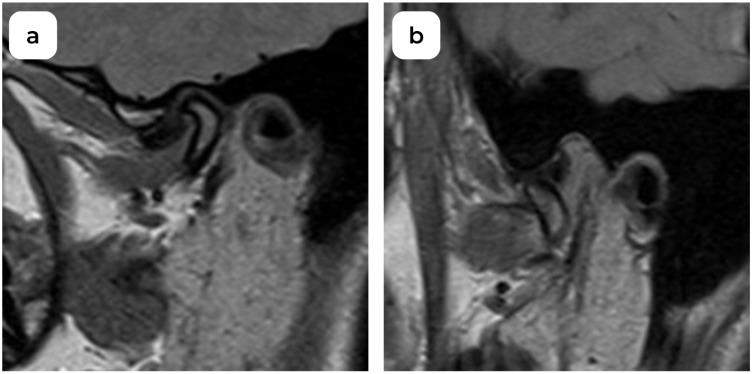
MRI oblique sagittal view left TMJ: (a) anterior disc displacement in closed-mouth acquisition; (b) open-mouth normal disc acquisition (DDwR).
